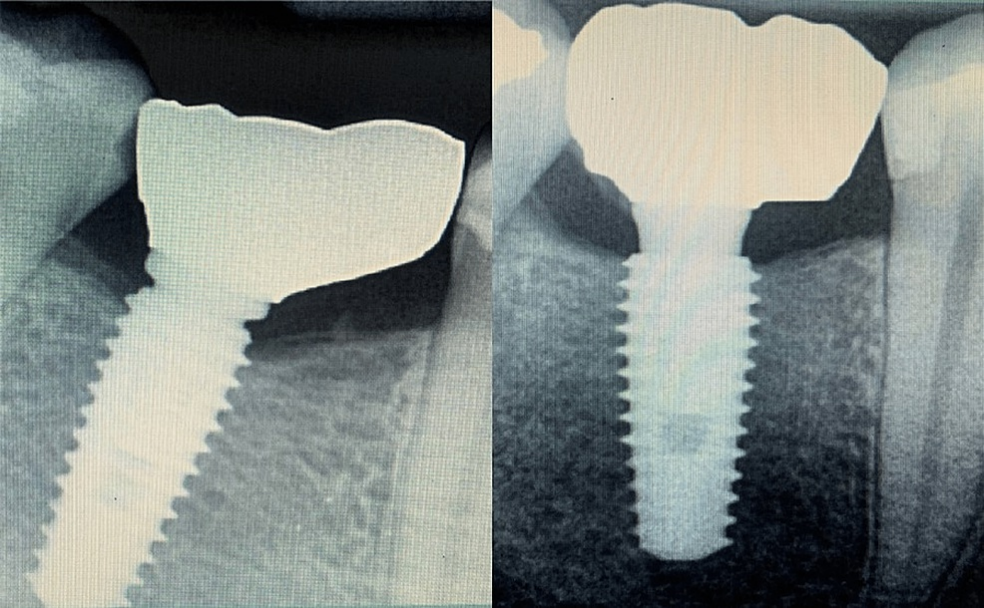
Radiographic evaluation of the final prostheses’ fitting over the implants
